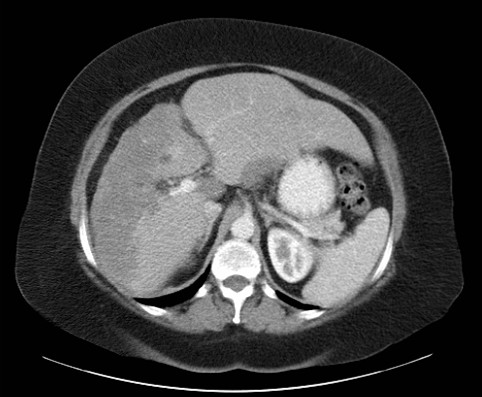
Abdominal computed tomography scan from 2002 showing a large tumour in the liver that was confirmed on biopsy as hepatic epithelioid hemangioendothelioma..
